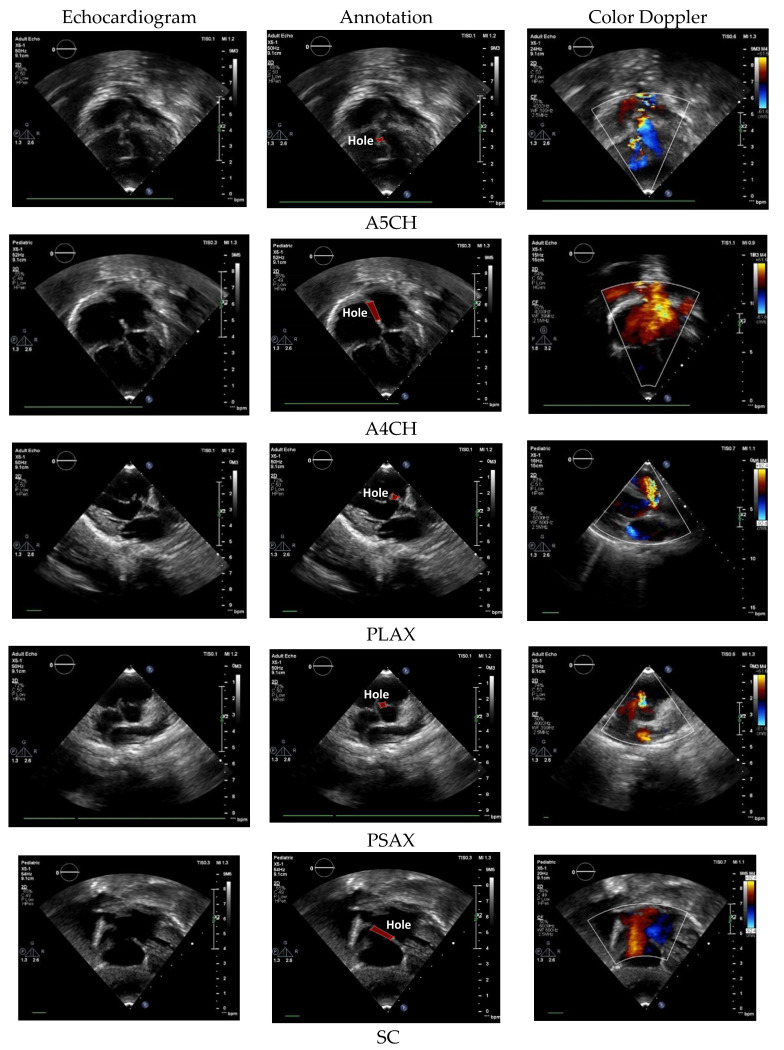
The US standard cardiac view of A4CH, A5CH, PLAX, PSAX, and SC with color Doppler echocardiography.
